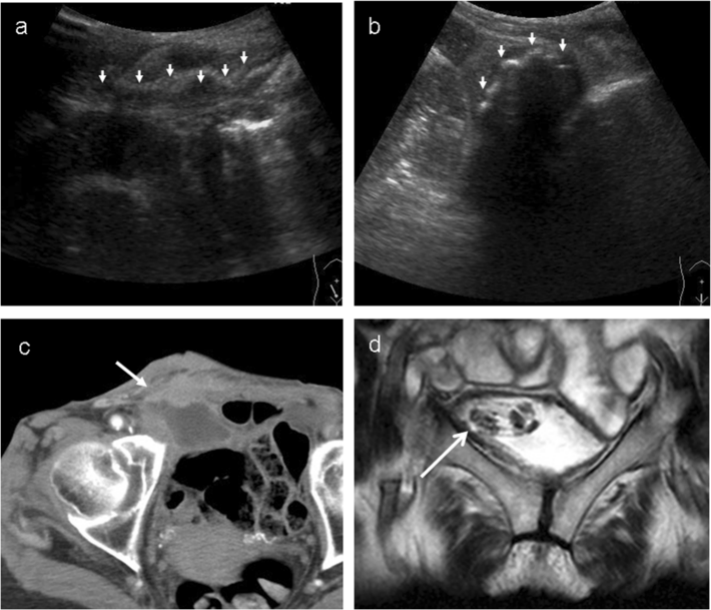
Image findings of mesh plug penetrated into the bladder. Abdominal ultrasound tomography detected (a) onlay mesh and (b) mesh plug. (c) Computer tomography showed high-density area (mesh plug) in the subcutaneous of right inguinal lesion. (d) Magnetic resonance imaging detected the mesh plug penetrated into the bladder.
